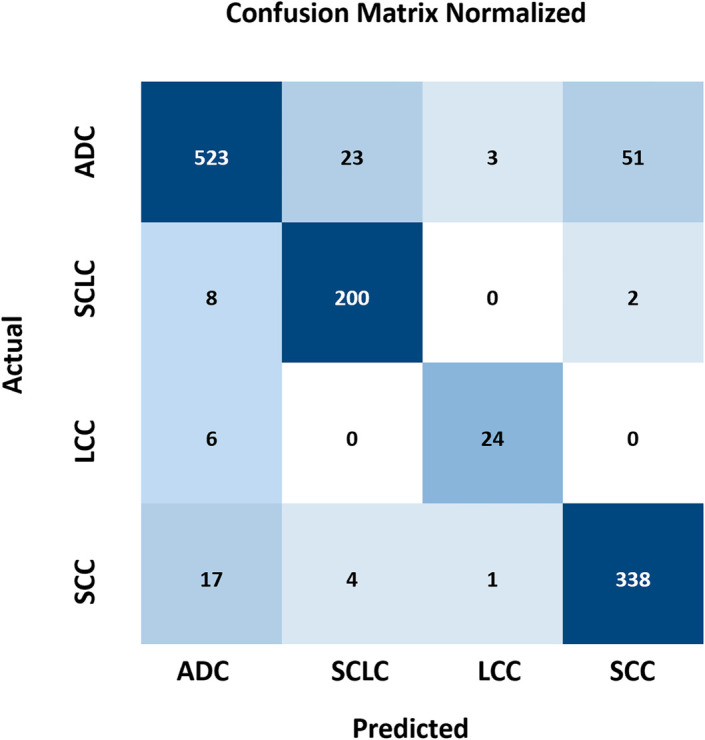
Lung cancer classification results on LUNG-PET-CT-DX.
